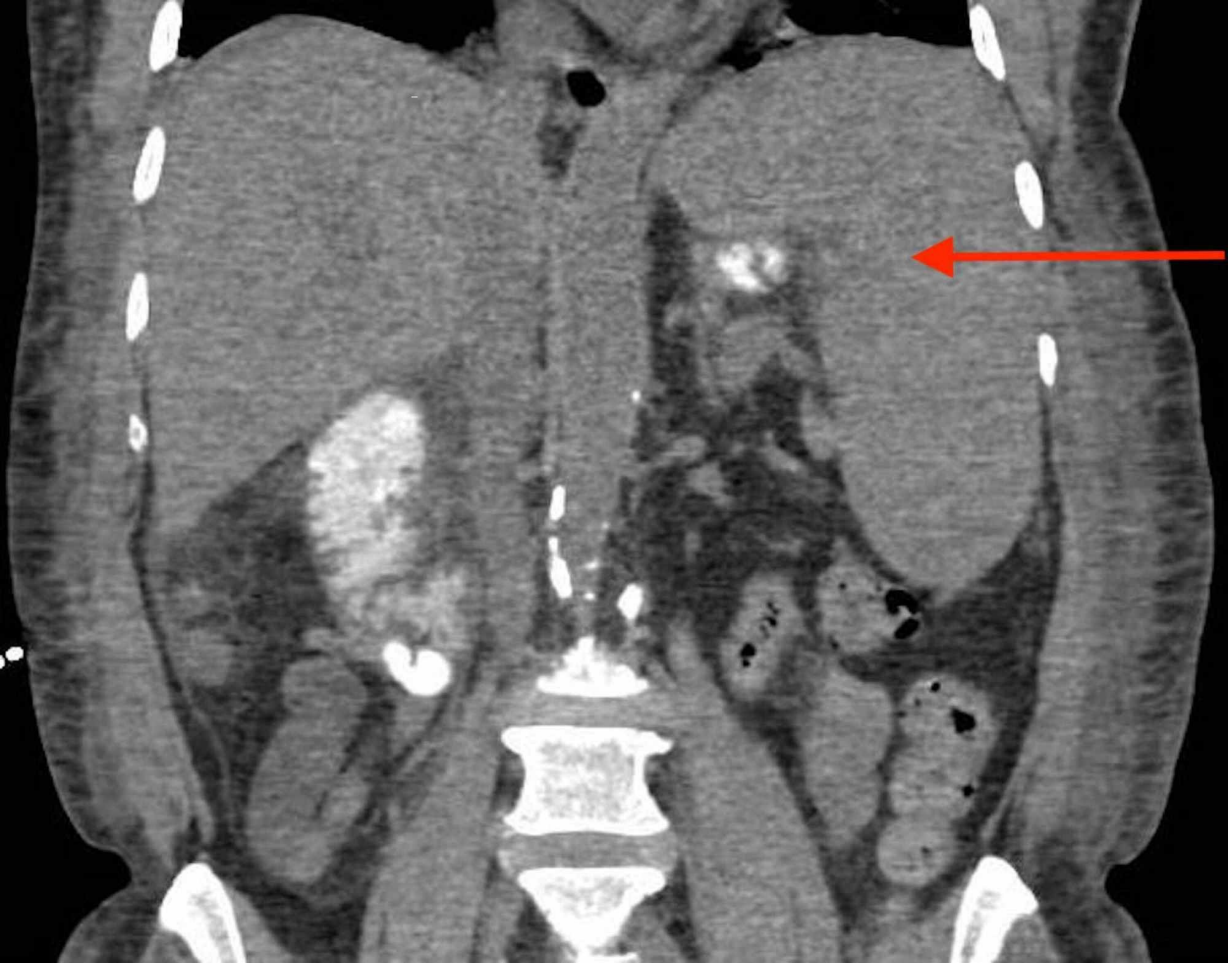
Coronal section of CT abdomen revealing splenomegaly of 17 cm (red arrow).
